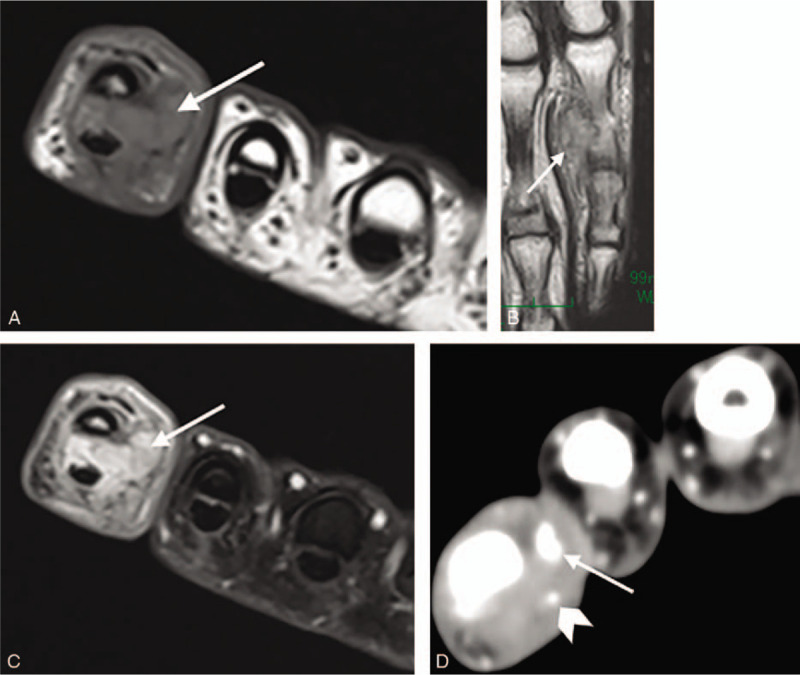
MRI T1-weighted axial view (A) demonstrating that the signal intensities of the lesion were homogenous hypointense (arrow). T2-weighted coronal view (B) demonstrating that the signal intensities of the lesion were heterogenous hyperintense (arrow). Contrast-enhanced T1-weighted axial view (C) demonstrating an enhanced T-shaped mass (arrow). Contrast-enhanced CT axial image (D) demonstrating that the mass was adjacent to the radial neurovascular bundle (arrowhead), and the surrounding calcifications were not continuous with the proximal phalanx bone (arrow). CT = computed tomography, MRI = magnetic resonance imaging.
